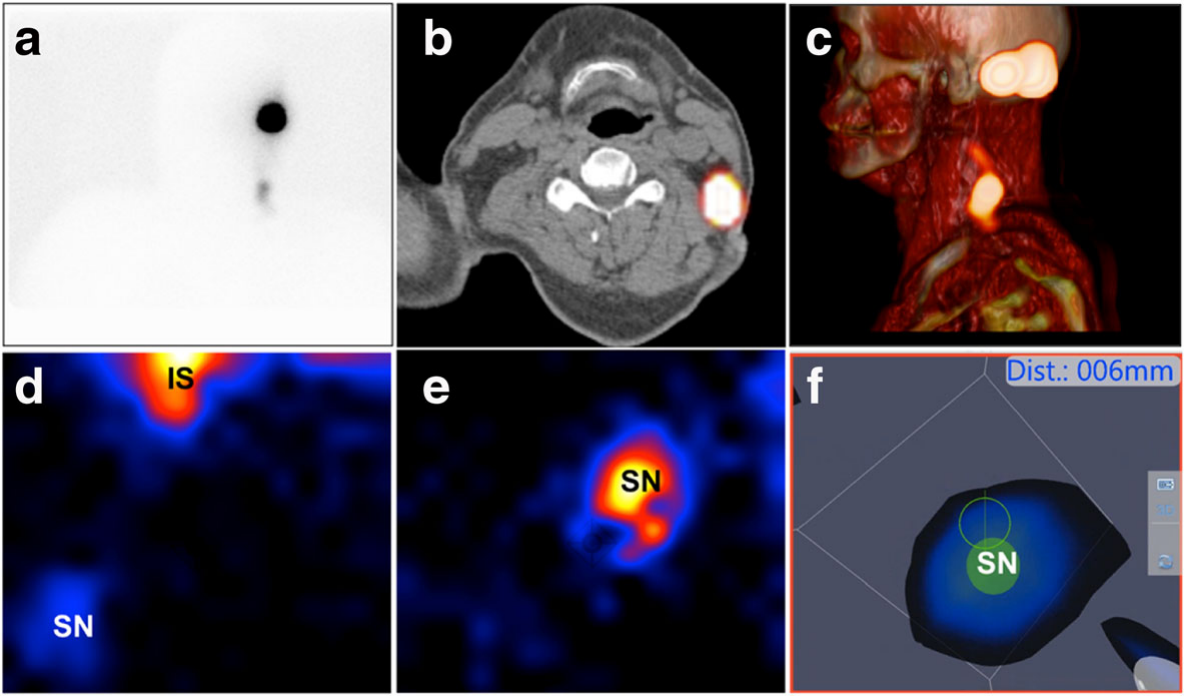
Overview of the acquired images. a Example of an anterior lymphoscintigram showing a clear sentinel node in the neck. b, c SPECT/CT imaging of the patient shown under a allowed placement of the hotspot in its anatomical context with the sentinel node being located in level V. The fused SPECT/CT images provided the surgeon with an anatomical roadmap for planning of the surgical procedure. d 2D mobile gamma camera image acquired in the operation room showing a sentinel node (SN) and the injection site (IS). e Zoom-in of the image shown in d. f A freehandSPECT scan was acquired and subsequently the gamma probe was navigated, in augmented reality, to the sentinel node as seen in the freehandSPECT scan
